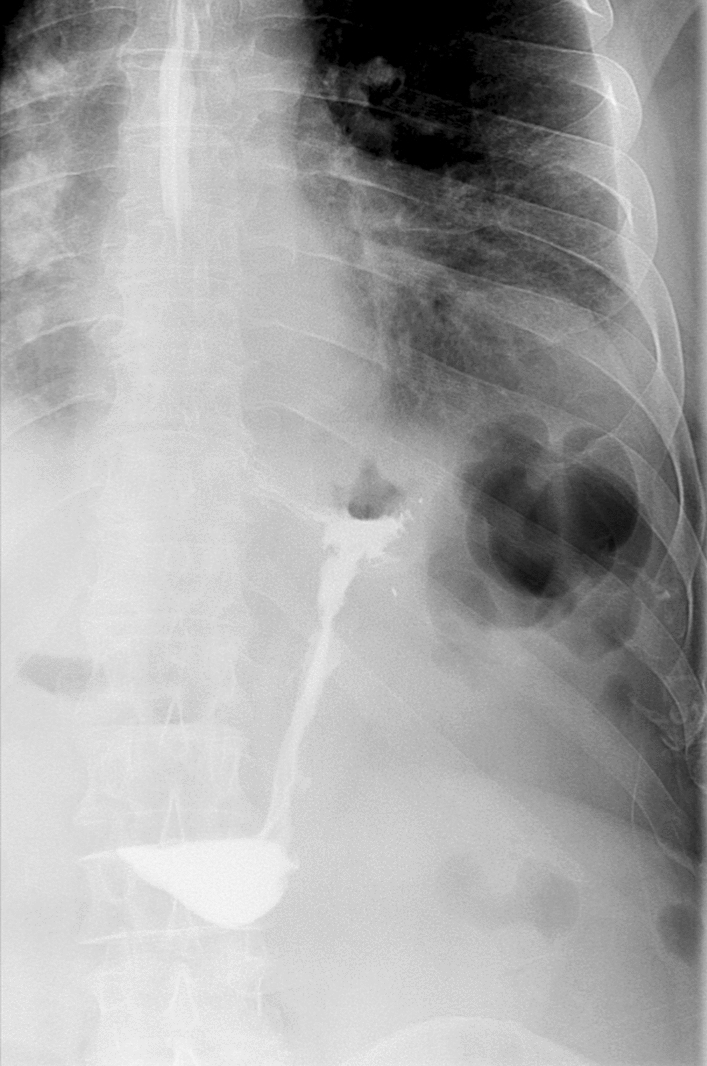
Swallow X-ray after the ESG procedure
